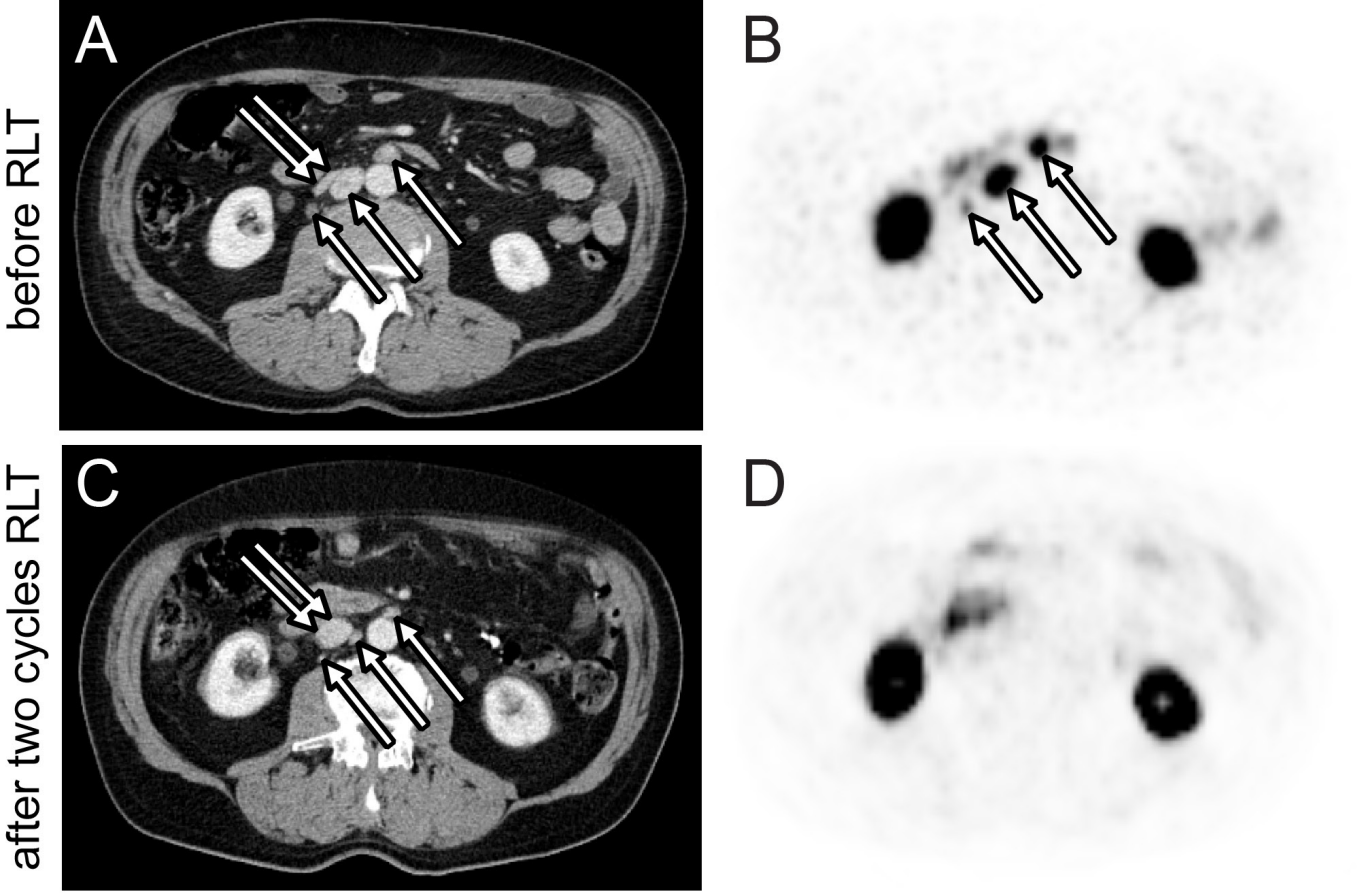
Response after two cycles of 6.0 GBq ^177^Lu-PSMA-617 RLT Axial ^68^Ga-PSMA PET B. and D. and CT A. and C. images of the abdomen before (A and B) and after (C and D) two RLT cycles. Lymph node metastases demonstrate a >30% baseline to follow-up decrease in short axis diameter (A and C, arrows) and SUV_max_ (B, arrows). Compression of the inferior vena cava by lymph node metastases at baseline (A, double arrow) was resolved at follow-up (C, double arrow).
